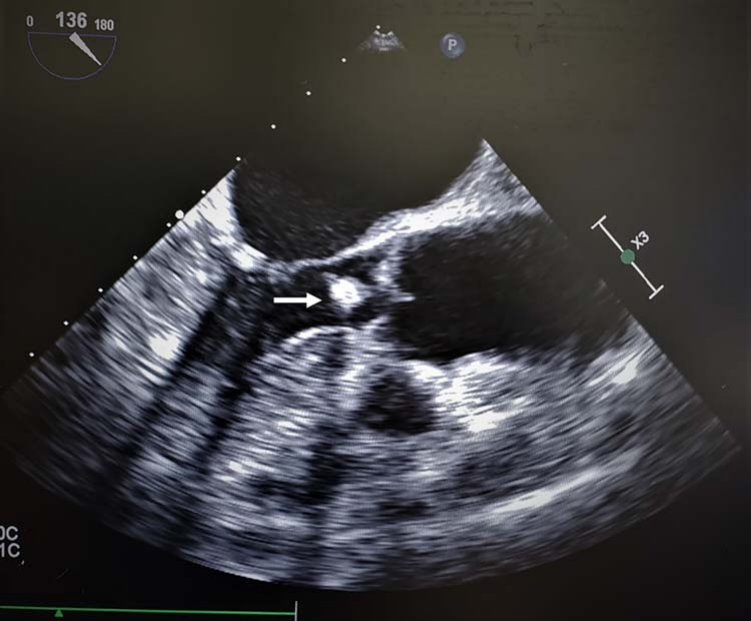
Transesophageal echocardiogram of cardiac amorphous tumor within the LVOT; intraoperative TEE revealed similar findings as a TTE in the outpatient setting; the hyperechoic sphere-like lesion can be identified within the LVOT in the mid-esophageal bicaval view (white arrow); the mass was mobile and nonobstructive to the LVOT; the attachment site was not visualized clearly.
